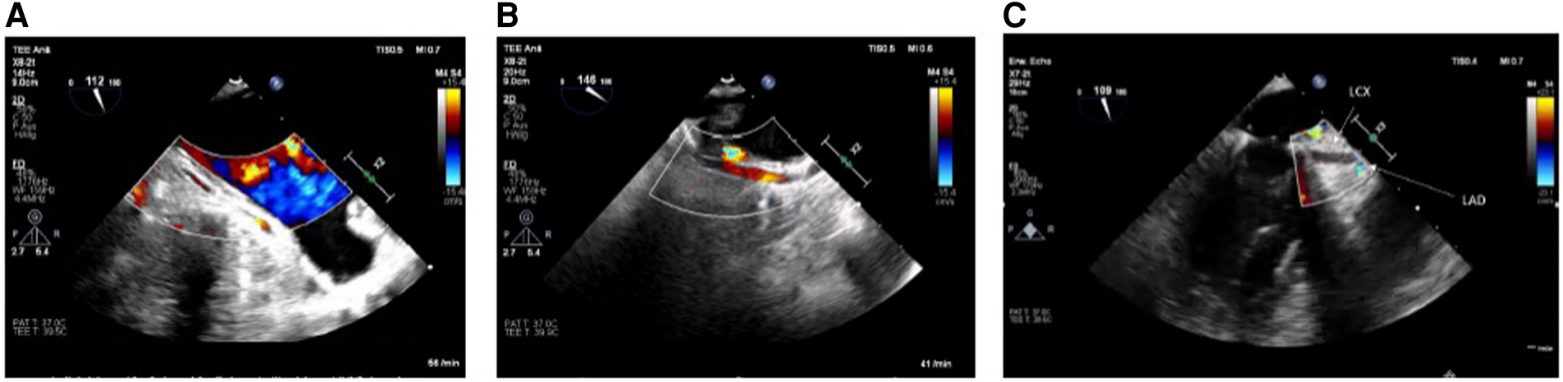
(A) LCX in long axis without flow in the midsegment through suture occlusion (TOE view: modified mid-esophageal aortic valve long axis view according to ender et al. (35) (ME AV LAX) with color flow Doppler, Nyquist limit <30 cm/s). (B) As a contrast: inconspicuous longitudinal view of a prominent LCX (TOE View: modified Mid-esophageal long axis view (mod. ME LAX) with color flow Doppler, Nyquist limit <30 cm/s. (C) Prominent LCX in long axis without flow detection in the proximal third and without the possibility of echocardiography imaging the LCX in the full length due to an estimated occlusion to be expected further peripherally [TOE view: modified mid-esophageal two chamber view (ME 2CH) with color flow Doppler, Nyquist limit <30 cm/s].
